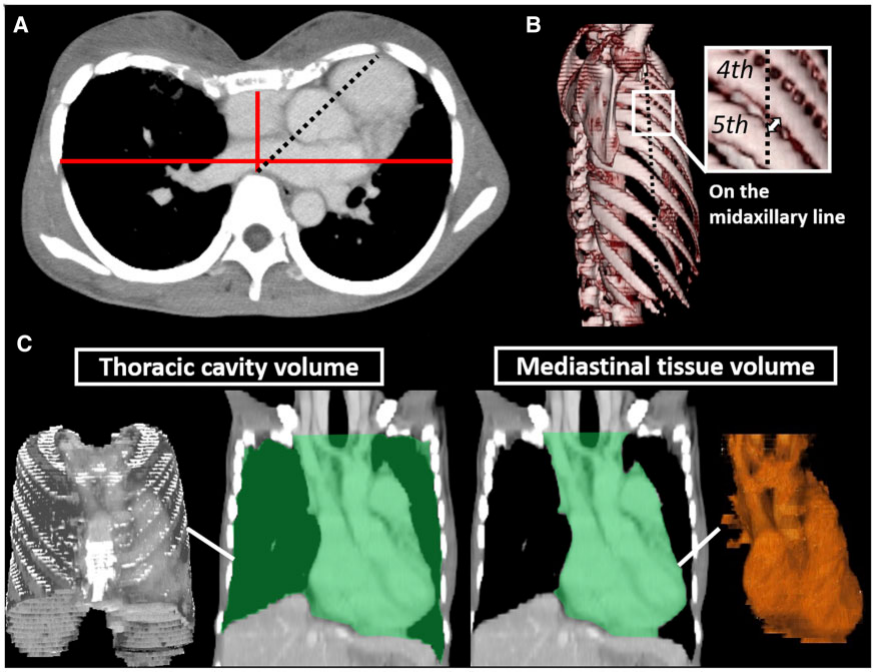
Assessment of the recipients’ physical traits on preoperative computed tomography. (A) The Haller index was calculated as the ratio between the maximum transverse diameter (the horizontal distance inside the ribcage) and the minimum anteroposterior diameter (the shortest distance between the vertebrae and the sternum); the horizontal and vertical red lines show these diameters, respectively. A mediastinal shift is diagnosed when there is a large gap of a >30° angle between the midsagittal line and the black dotted line joining the anterior aspect of the vertebral body and the centre of the aortic valve. (B) The fourth intercostal length (white arrow), which is measured vertically to the fifth rib on the midaxillary line (black dotted line). (C) Thoracic mediastinal-occupying ratio on three-dimensional computed tomography, which was measured by the formula: (mediastinal tissue volume/thoracic cavity volume) × 100 (%). The light green areas represent extracted areas.
